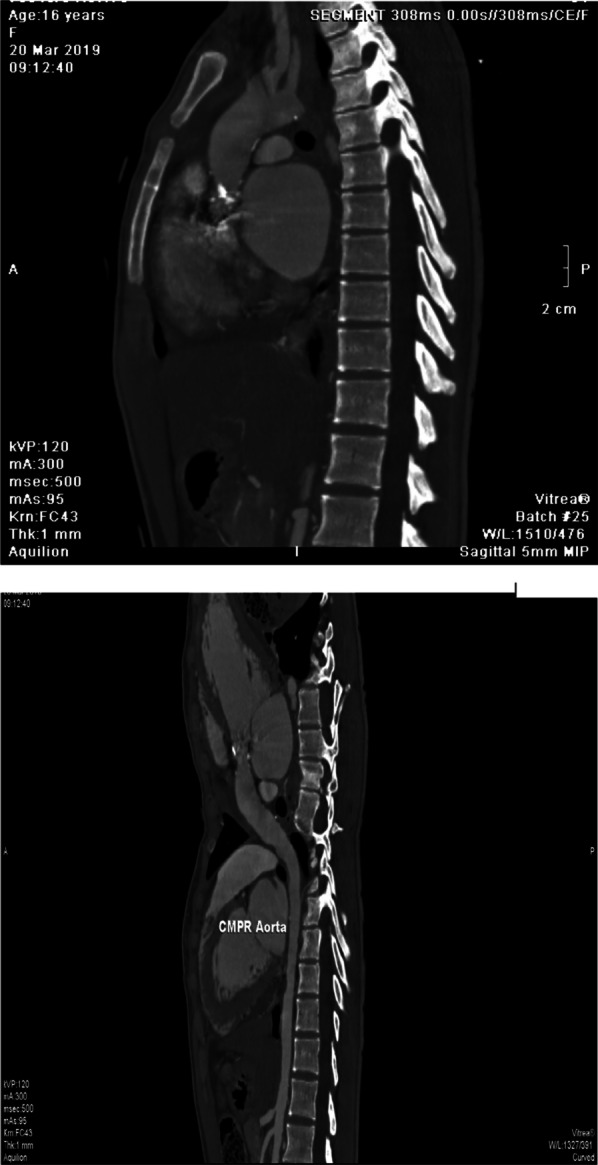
CT aortogram showing significant narrowing of aorta
